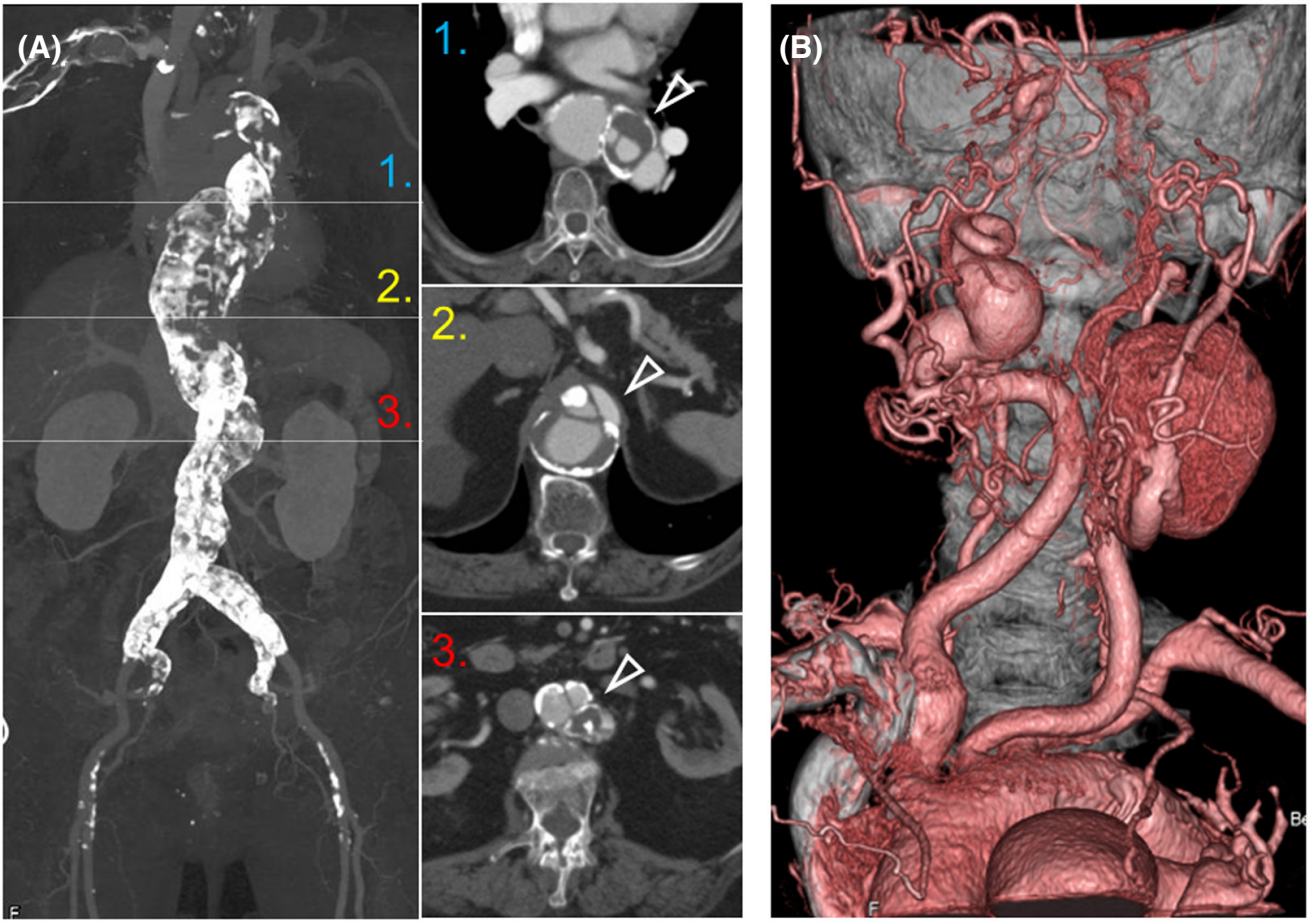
CT findings of the present case. (A) The severely calcified thoracoabdominal aorta image (left panel) with corresponding contrast‐enhanced CT images (right panels). Arrowheads indicate aortic dissections. (B) Three‐dimensional CT angiography. The right and left internal carotid arterial aneurysms were 22 and 56 mm in maximum diameter, respectively.
